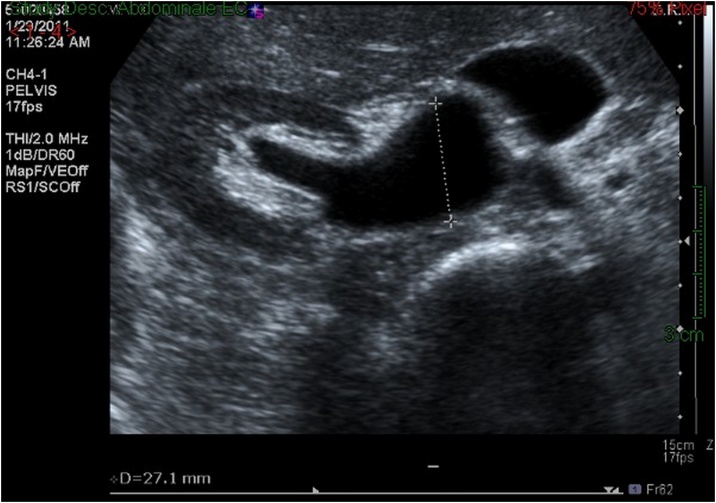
Renal ultrasound of patient 1 showing severe right-sided hydronephrosis.
